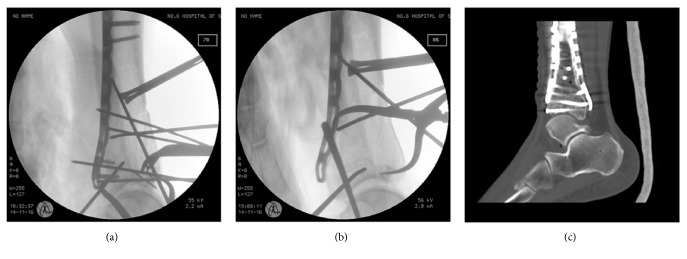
(a) The posterior column moved forward and the reduction was unsatisfactory. (b) After reduction of the anterior column, the posterior column was restored and the congruency of the ankle joint was satisfactory. (c) CT showed that the articulation and congruency were restored.
